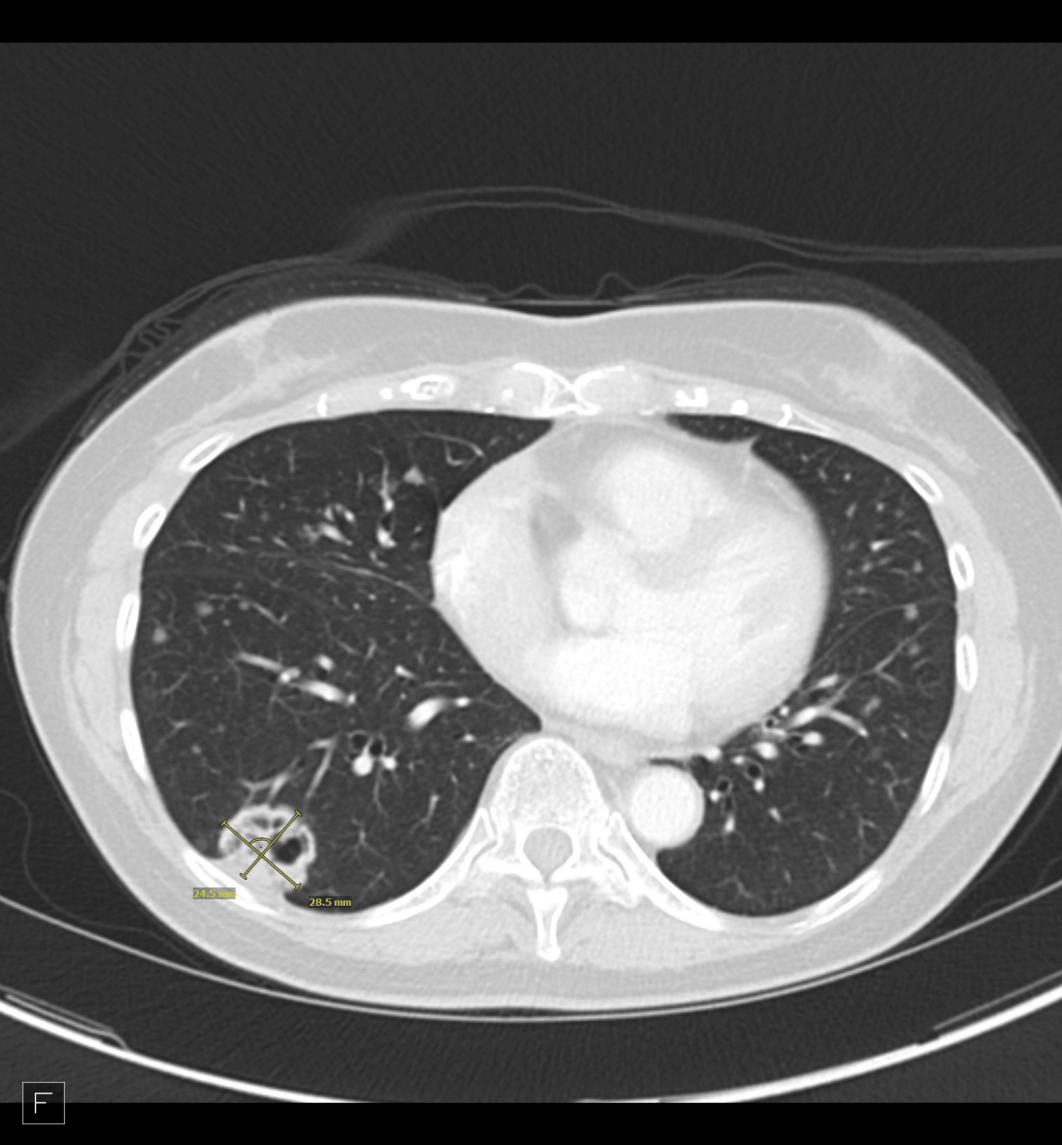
Contrast-enhanced axial chest CT demonstrating bilateral pulmonary nodules with a large cavitary lesion (measuring 29 × 25 mm) in the right lower lobe marked by a yellow X. CT: Computed tomography
